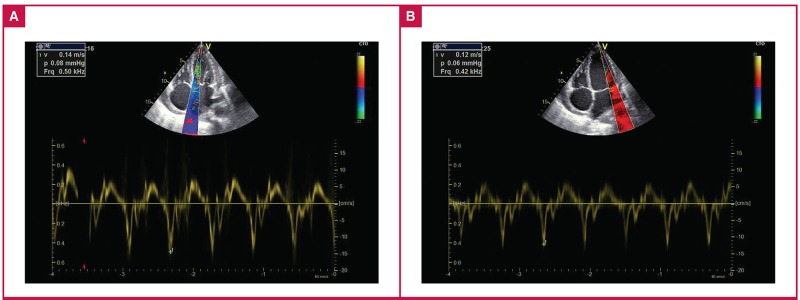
A. Tissue Doppler of the medial aspect of the mitral valve annulus demonstrating early diastolic tissue velocity of 0.14 m/s. B. Tissue Doppler of the lateral aspect of the mitral valve annulus showing early diastolic tissue velocity of 0.12 m/s. The lower tissue velocity on the lateral aspect is the opposite of the normal phenomenon (annulus reversus).
